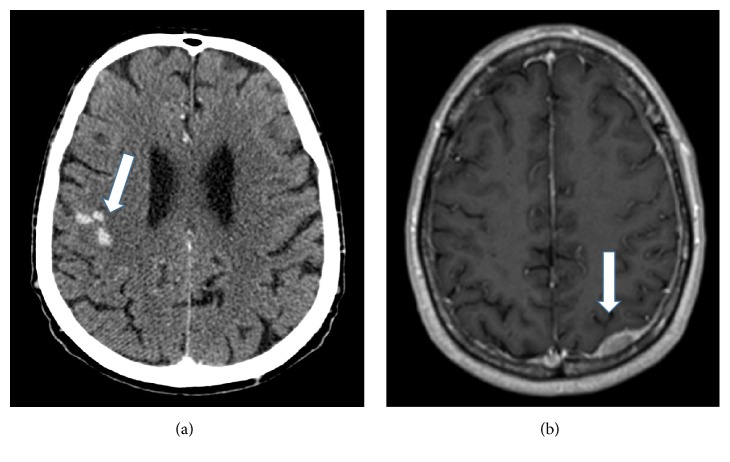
CT and MRI images of meningeal myeloma. (a) Contrast-enhanced CT; arrows: abnormally enhancing, leptomeningeal nodular lesions in the right frontal sulci (patient 4); (b) T1-weighted brain MRI after Gadolinium administration; arrows: abnormally enhancing dural lesion to be differentiated from subdural hemorrhage (patient 11).
